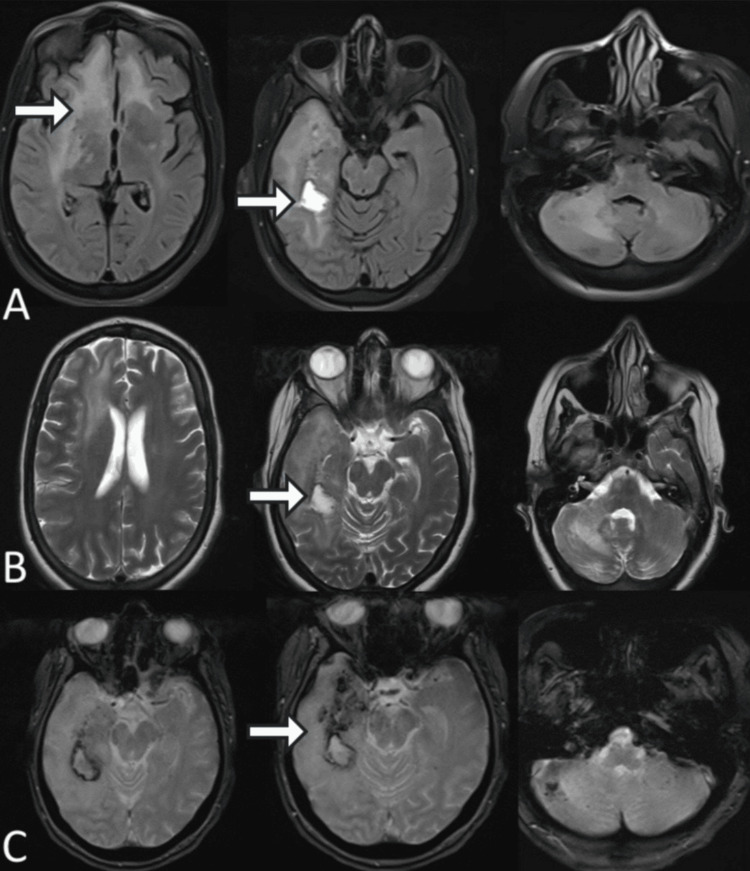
Follow-up brain MRI on day 15 showing marked radiological improvement (A) Axial FLAIR-weighted images demonstrate a marked reduction in the extent and signal intensity of previously described bilateral cortical-subcortical lesions involving the frontal, insular, and temporal regions, with significant regression of surrounding vasogenic edema (white arrows). (B) Axial T2-weighted images confirm interval improvement with decreased lesion size and reduced mass effect, with residual signal abnormalities in the temporal region (white arrows). (C) Susceptibility-weighted imaging (SWI)/T2-weighted images show a reduction in the number and extent of previously observed punctate hypointense foci, consistent with resolving microhemorrhages (white arrows).
